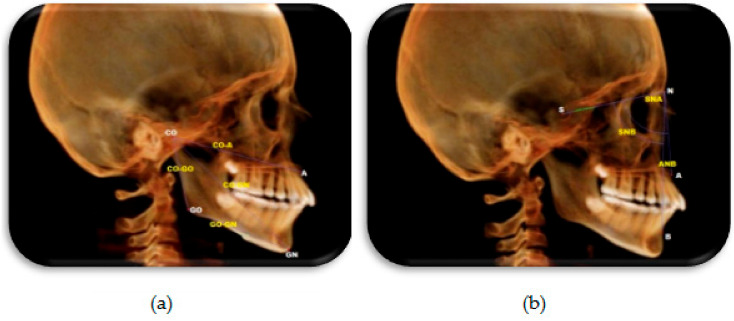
Constructed lateral cephalometric radiograph showing skeletal landmarks and measurements used in the study. (a) Sagittal measurements. (b) Angular measurements.
